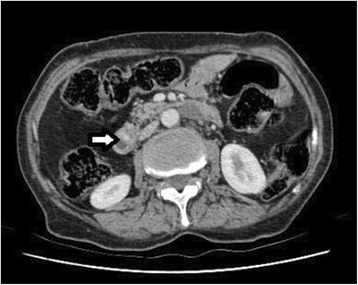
Contrast enhanced computed tomography of abdomen showing the small enhancing periampullary neuroendocrine tumor (arrow)
